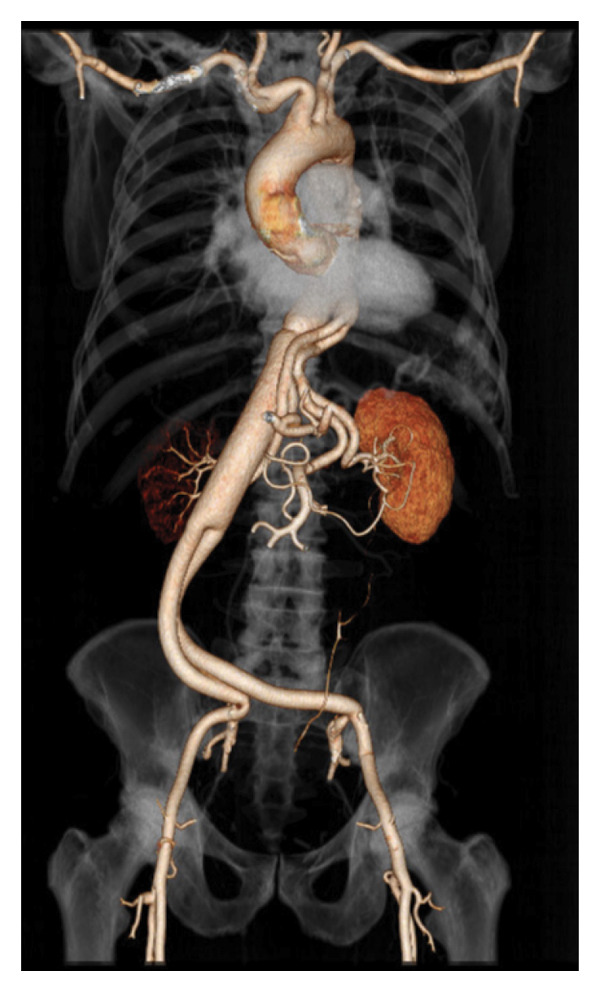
CT angiogram of abdomen and pelvis with angiographic reconstruction demonstrating infrarenal aortobiiliac endograft.
